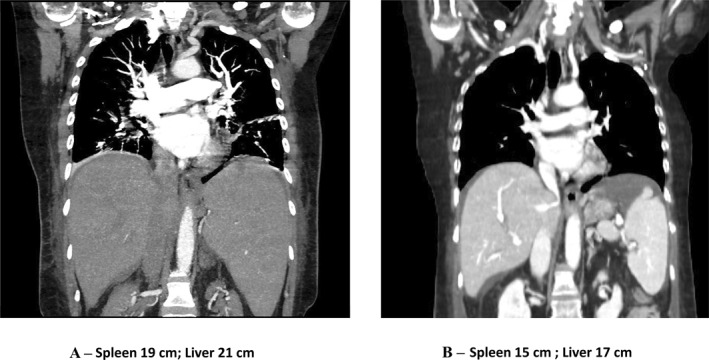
Evolution of the computed tomography scan of the patient's liver and spleen (A, B).
